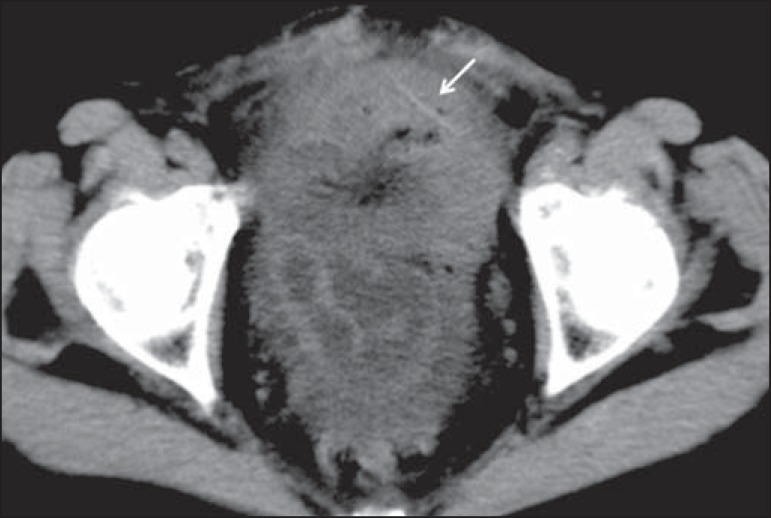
A 83-year-old female patient. A CT scan of the abdomen showing
